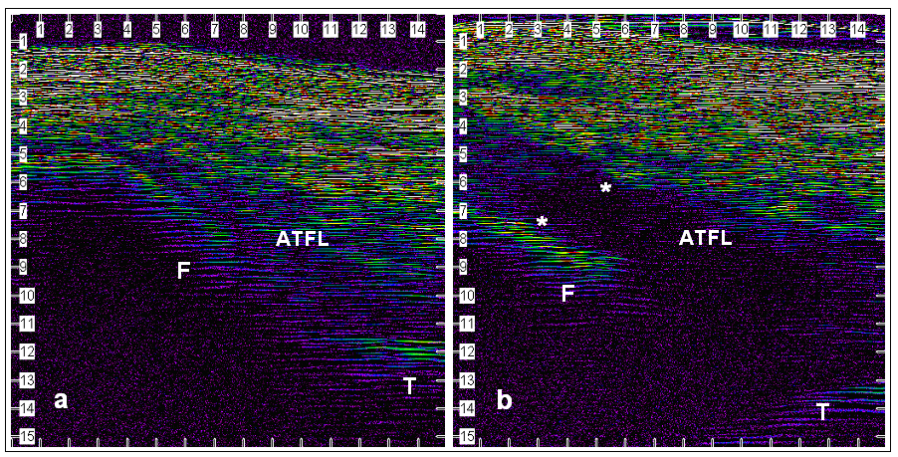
Ultrasound images of lateral ankle. (a) Un-injured control. (b) Grade I sprain 7 days post injury. The hypoechoic zone between the markers (*) indicates an area of soft tissue swelling. F = Fibula. T = Talus. ATFL = Anterior talofibular ligament. [Scale = mm]
